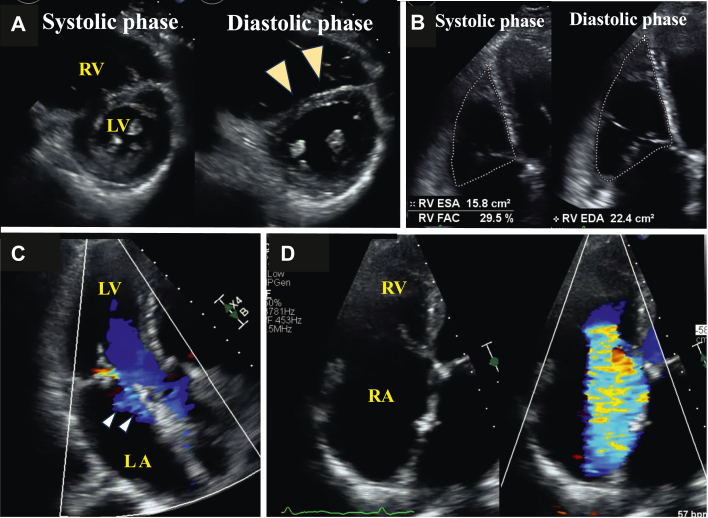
Transthoracic Echocardiography on Admission (A) The ventricular septum was compressed toward the LV side during diastole (arrowheads). (B) RV dysfunction. (C) Mild mitral regurgitation. (D) Torrential tricuspid regurgitation with leaflet tethering. LA = left atrium; LV = left ventricle; RA = right atrium; RV = right ventricle.
